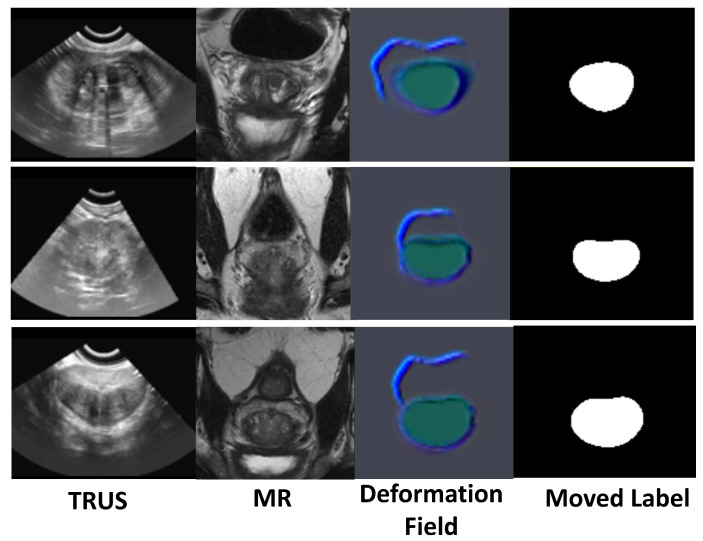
Experimental results: TRUS and MR images with corresponding deformation field and moved label.
